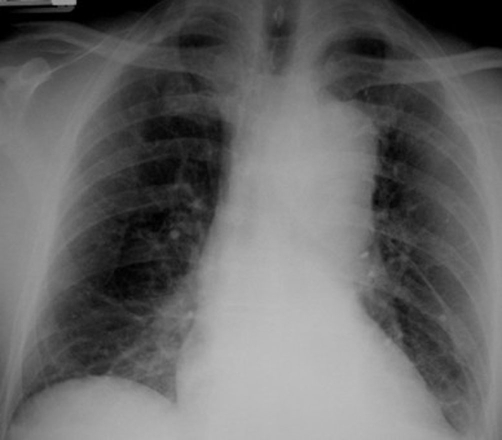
AP chest X-ray on admission.
